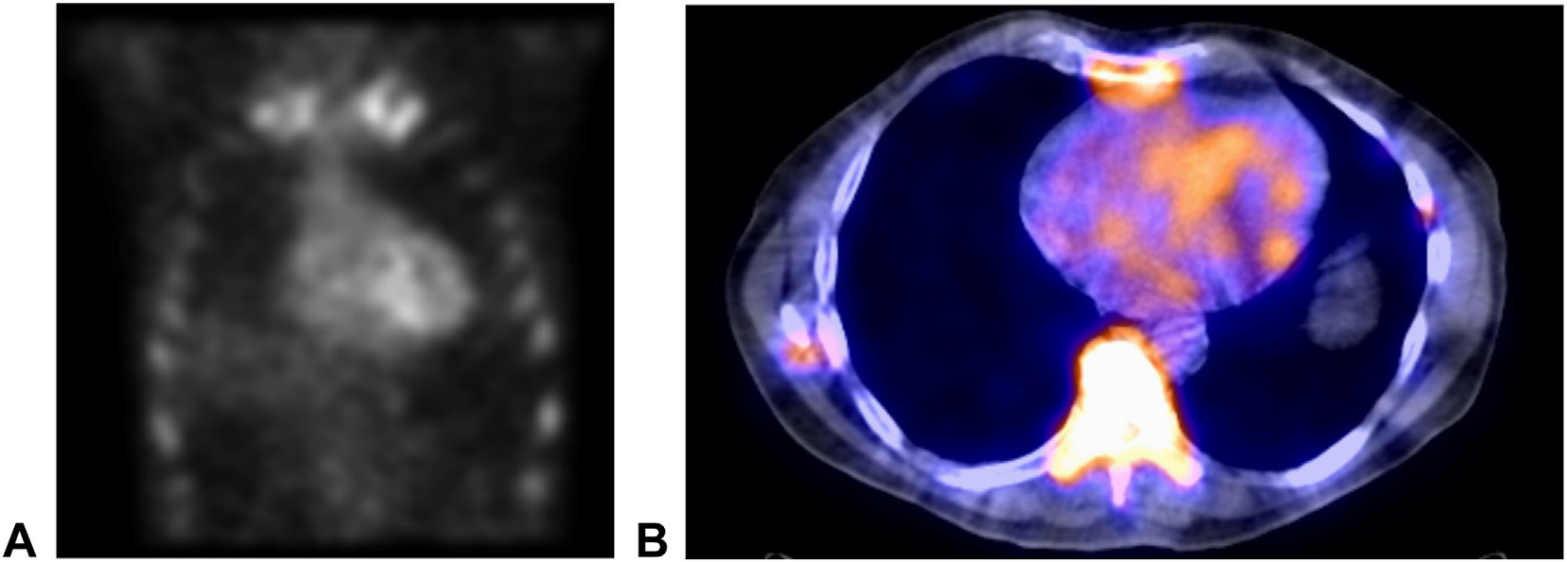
Technetium-99m pyrophosphate scan A with SPECT-CT (single-photon emission computed tomography [SPECT] combined with low-dose non-contrast computed tomography [CT]) B demonstrating increased radiotracer uptake involving all four walls of the left ventricle, supporting the diagnosis of cardiac amyloidosis.
